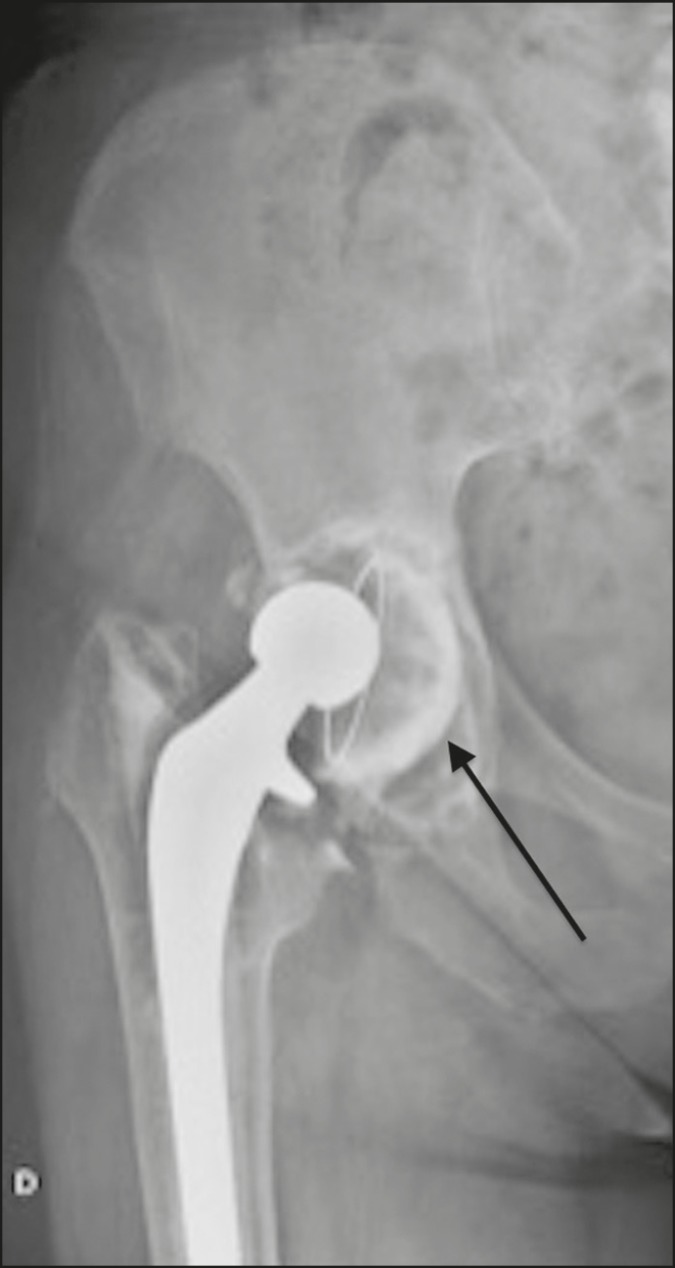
Anteroposterior X-ray of the right hip. Total right hip arthroplasty with vertical orientation and dislocation of the right acetabular component of the prosthesis (arrow).
